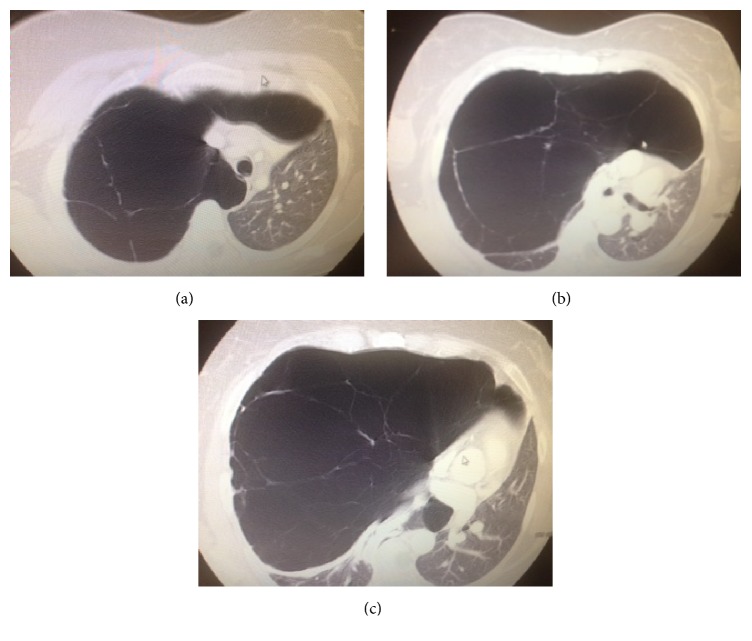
Chest CT scan depicts severe bullous changes, occupying approximately 85% of the right lung and displacing the mediastinum and left lung laterally. Smaller bullous changes are noted in the remaining aerated portion of the right lung. Some portions are significantly compressed between the bullous changes and the aerated portion of the lung. The left lung is also significantly compressed but remains aerated.
